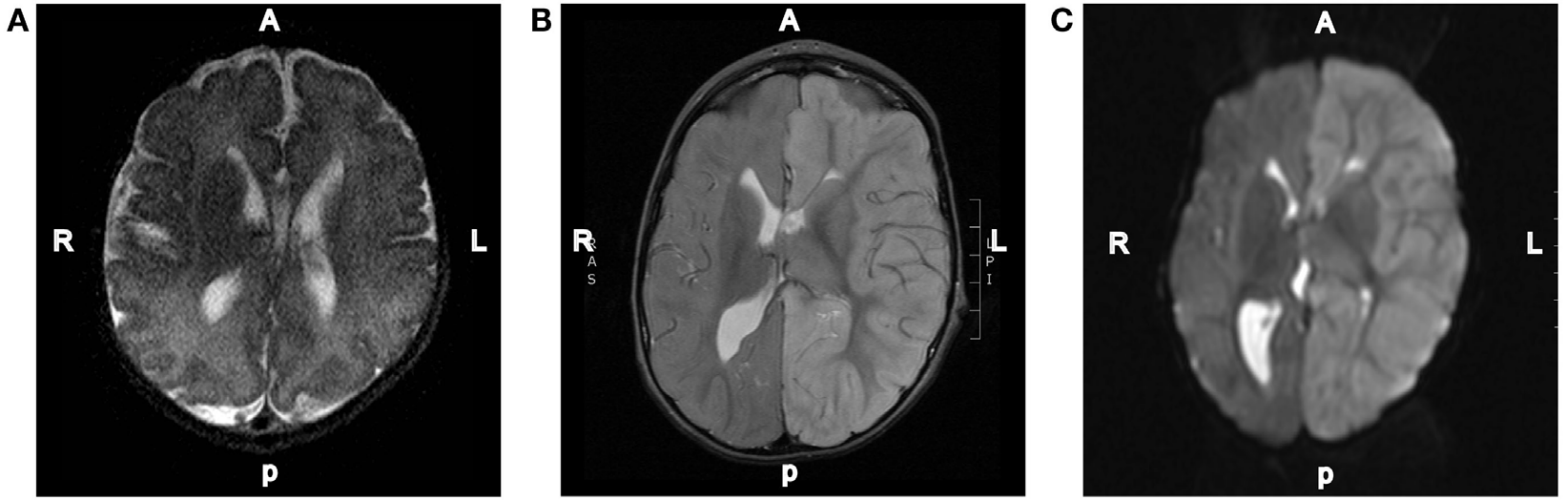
Representative MRI images. (A) T2-weighted axial brain MRI done of this patient at 3 days of age. (B) T2-weighted axial brain MRI done on day 2 of the hospital admission showing diffuse increased T2 signal intensity throughout the left hemispheric gray matter. (C) Diffusion-weighted axial brain MRI done on day 2 of the hospital admission showing diffuse left hemispheric cerebral edema.
